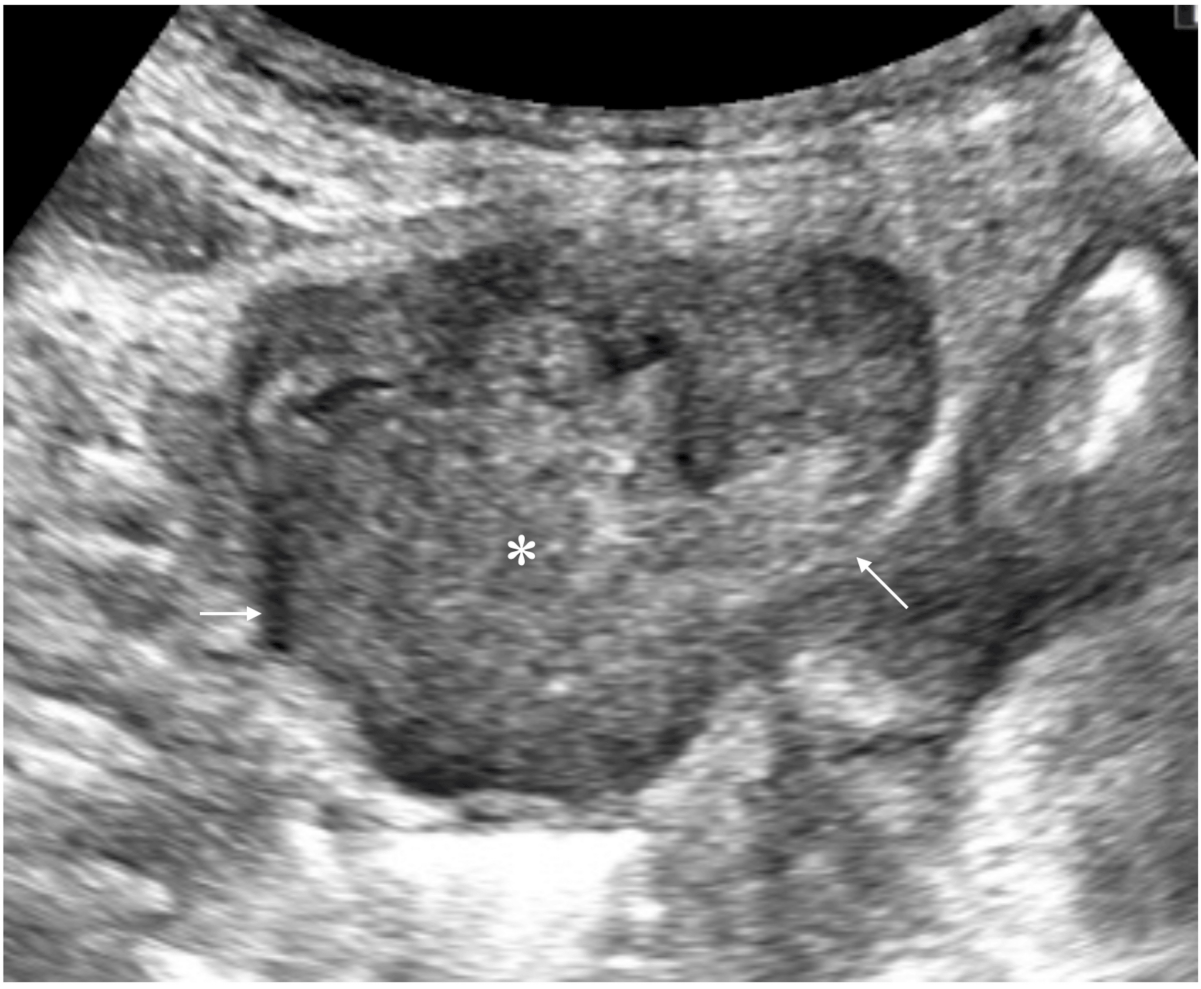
Ultrasound findings Ultrasound showed a well-circumscribed polygonal mass (arrows) with internal high echoes (asterisk)
